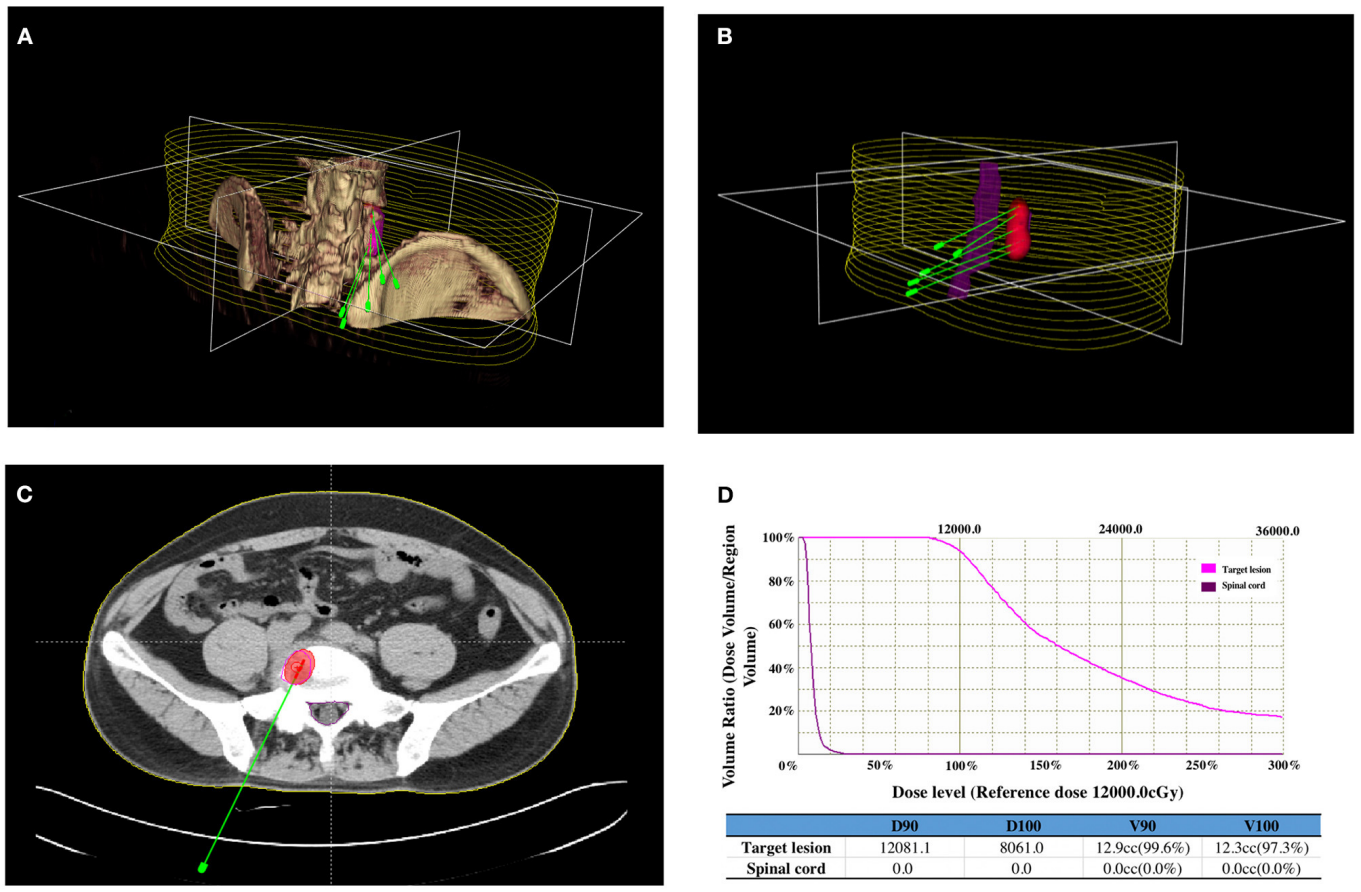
Iodine-125 (^125^I) brachytherapy treatment plan for bone metastases from breast cancer: (A) CT images of the lesion were reconstructed using a computerized treatment planning system (TPS), and the position of the brachytherapy applicators was calculated. (B) Three-dimensional image showing the relationship between the positions of the applicators, lesions, and spinal cord. (C) Two-dimensional image showing the irradiation dose (red area) of the ^125^I brachytherapy field, tumor contour (purple line), and spinal cord (green line). (D) Dose-volume histogram indicating the prescribed dose to the lesion and the dose received by the spinal cord.
